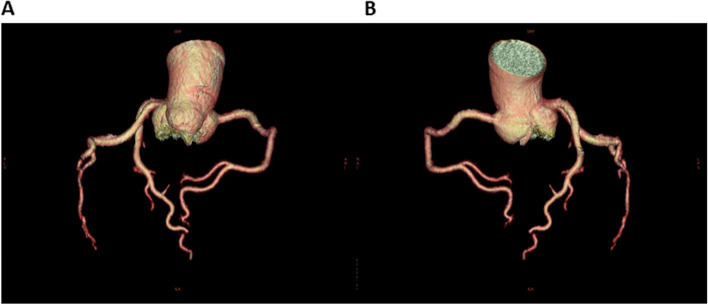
Computed tomography (CT) angiography at 7 months after onset of first headache. Coronary computed tomography (CT) angiography 7 months after onset showed no significant vasoconstriction in any coronary artery. Part (A) shows right anterior oblique (RAO) artery images, and part (B) shows left anterior oblique (LAO) artery images
